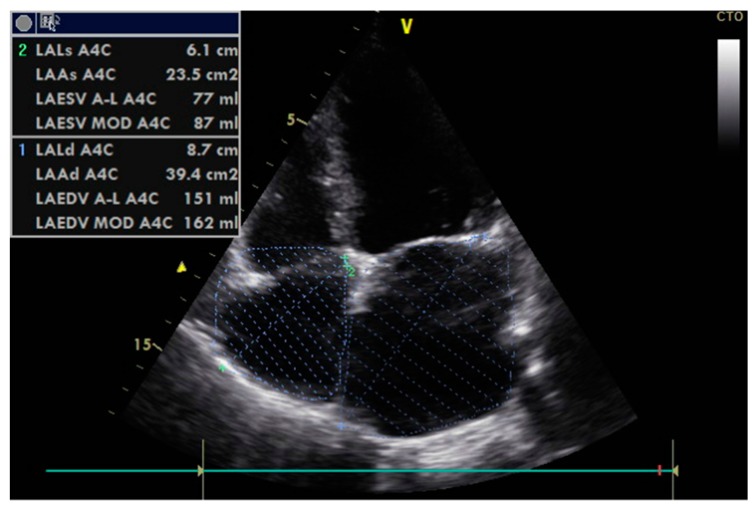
Echocardiographic measurement of left (and right) atrial volume by disks method. V: specific echocardiographic parameter (it does not mean a measurement data).
